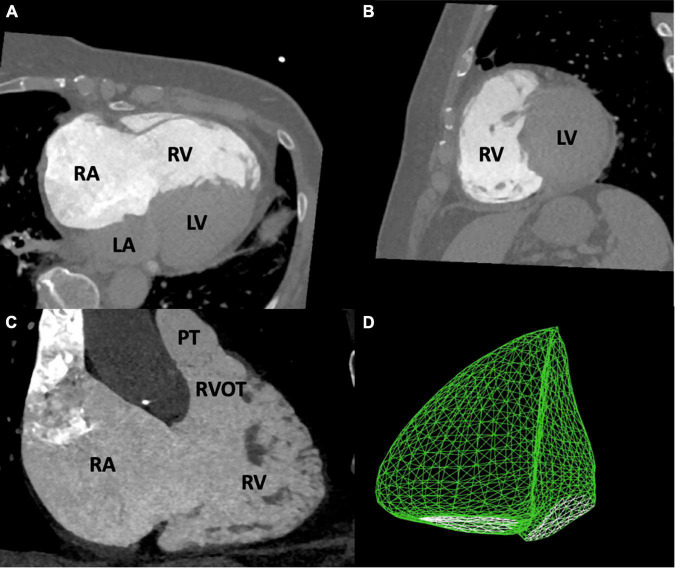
Anatomy of the right ventricle. This figure shows images of the right heart derived from multidetector computed tomography (A–C) and three-dimensional echocardiography (D). The RV is crescent shaped in an axial (A,C) and triangular in a lateral view (B). LA, left atrium; LV, left ventricle; PT, pulmonary trunk; RA, right atrium; RV, right ventricle; RVOT, right ventricular outflow tract.
